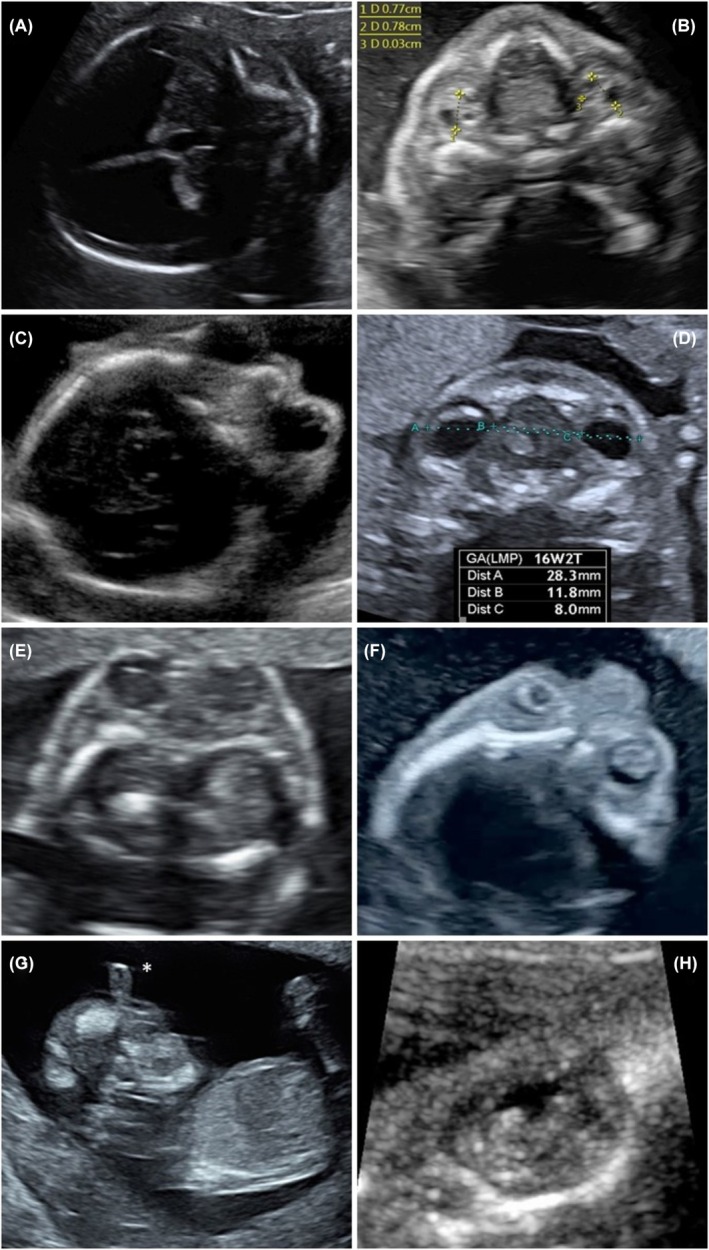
Examples of ocular findings in ultrasound. (A) anophthalmia, (B) microphthalmia, (C) exophthalmos, (D) hypertelorism, (E) hypotelorism, (F) cataract, (G) cyclopia (with proboscis*), (H) retinal detachment.
